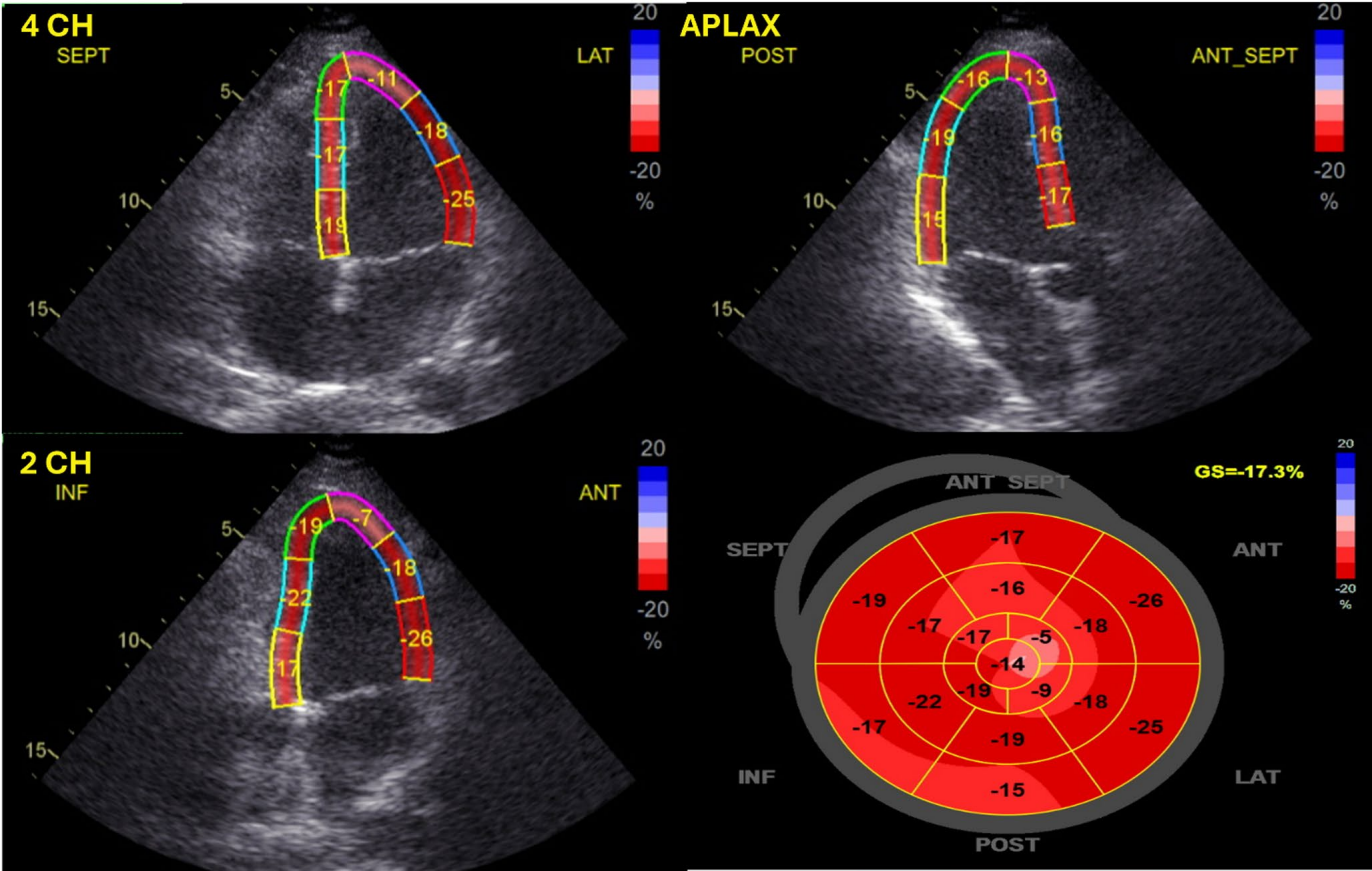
Speckle-tracking echocardiographic strain analysis of a representative patient from the study cohort. Apical four-chamber (4CH), three-chamber (APLAX), two-chamber (2CH), views are presented, each displaying regional peak systolic longitudinal strain. The bull’s-eye plot integrates data from all 18 LV segments derived from these views and provides the global longitudinal strain (GLS) value. In this case, GLS is reduced, reflecting subclinical left ventricular systolic dysfunction
